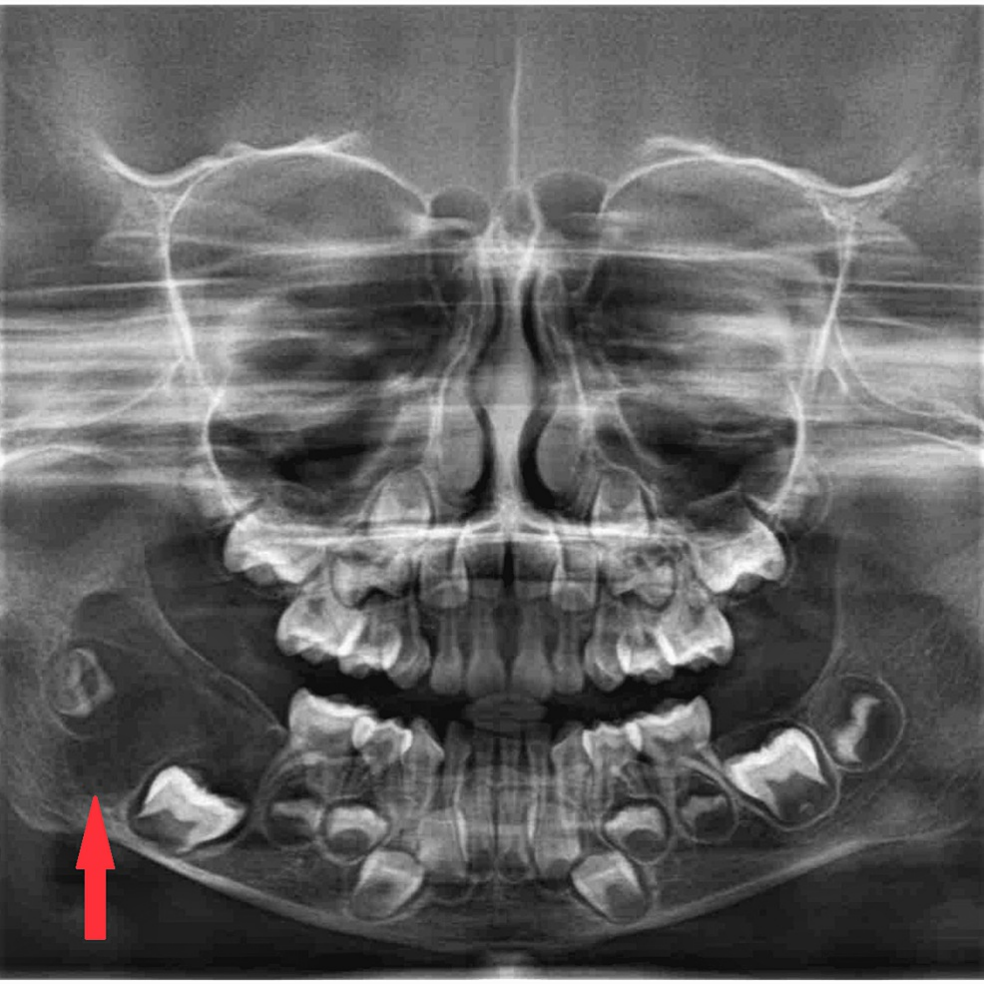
Orthopantomogram Orthopantomogram revealed a  well-defined multilocular radiolucency located at the angle region of the right mandible.
